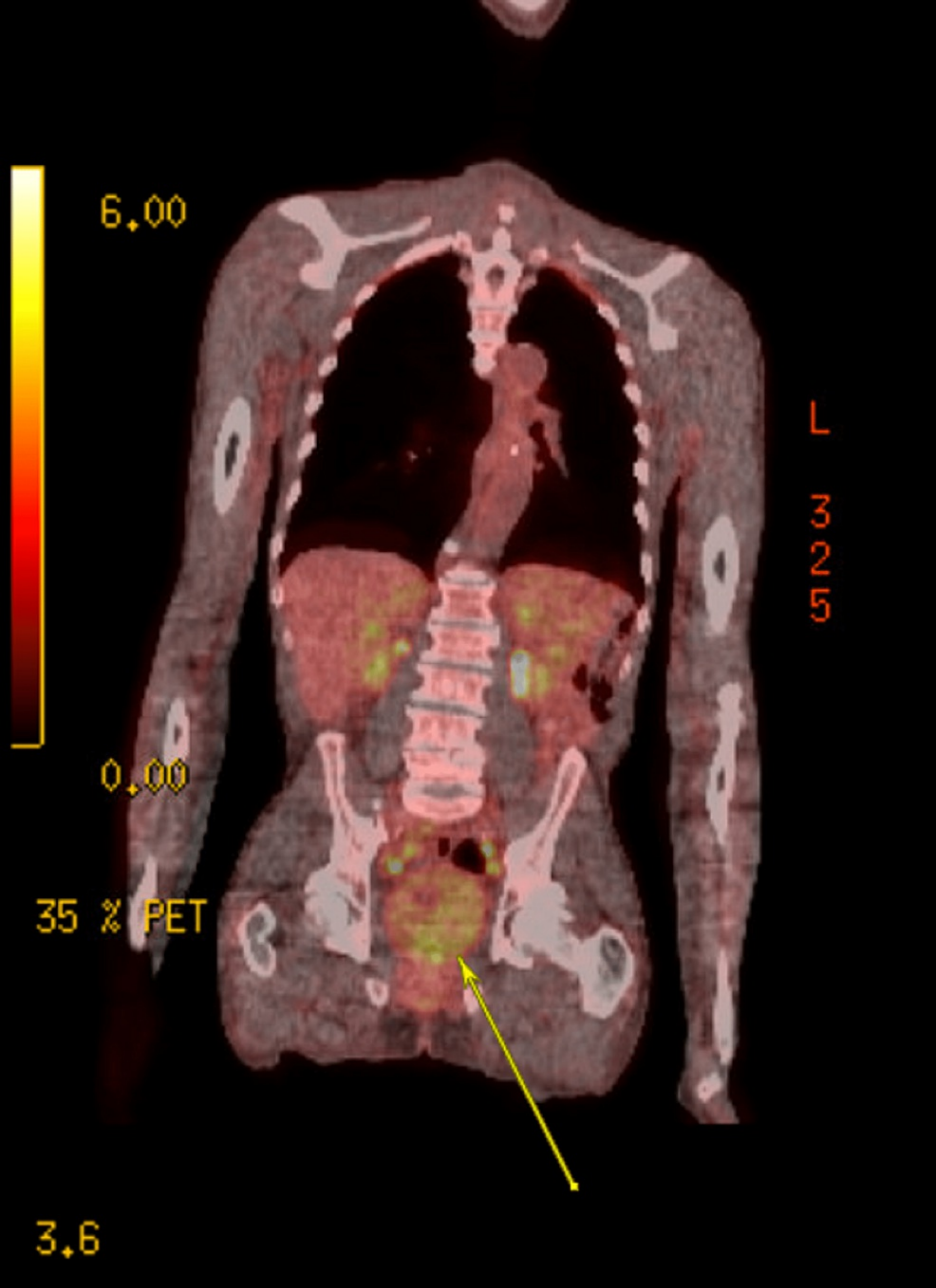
PET-CT scan demonstrating the extent of disease within the prostate.
